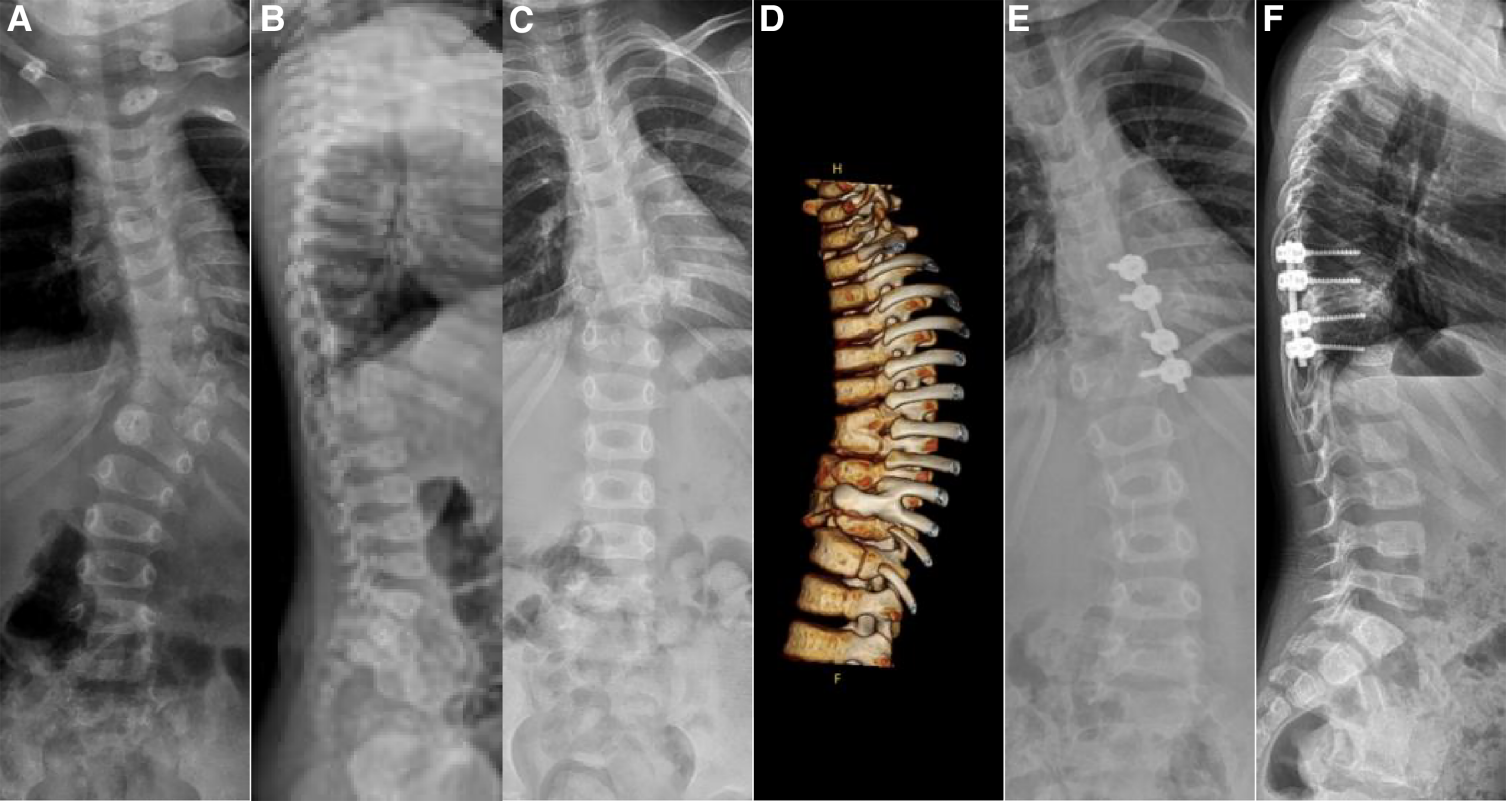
Case 2, female, 6 months old. Preoperative orthogonal (A) and lateral (B) x-ray showing the left hemivertebrae of T10. Intraoperative hemivertebra resection was performed *via* the posterior approach. After 4 years of follow-up, orthogonal x-ray (C) and lateral computer tomography (D) showed good correction of coronal scoliosis, but sagittal kyphosis was aggravated, and a second internal fixation revision surgery was performed. Post-revision orthogonal (E) and lateral (F) x-ray showed good correction of the coronal and sagittal deformity.
